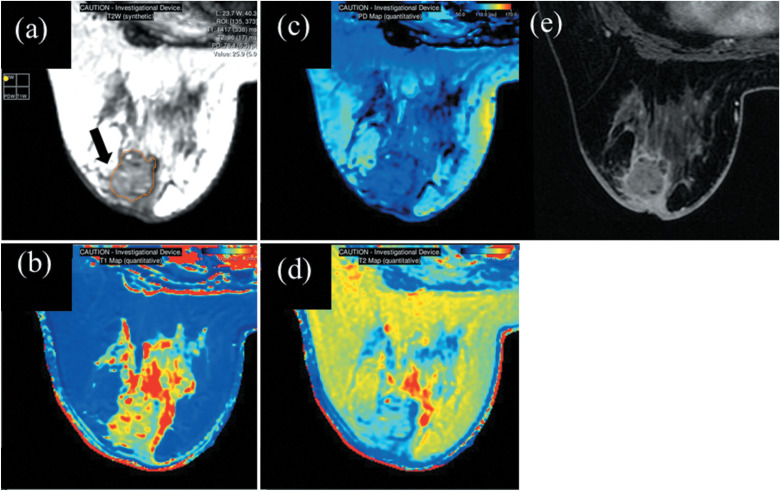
A woman in her 40s with HER-2 enriched breast cancer (cT4bN2M0 cStageIIIB). Pre contrast SyMRI (synthetic T2-weighted image: a, T1 map: b, PD map: c, T2 map: d) and DCE-MRI (e) show a lobulated mass enhancement with irregular shape and rim enhancement in the left breast (arrow). A ROI to obtain quantitative values in SyMRI was manually drawn to encompass as much of the abnormality as possible and stay within the border of the breast lesion using DCE-MRI as a reference and placed in the synthetic T2-weighted image. The T1, T2, PD, SD of T1, SD of T2, and SD of PD of the lesion obtained from SyMRI using ROI are1417 ms, 96 ms, 78.3 pu, 338, 17, and 6.3, respectively. DCE, dynamic contrast-enhanced; PD, proton-density; SD, standard deviation; SyMRI, synthetic MRI.
